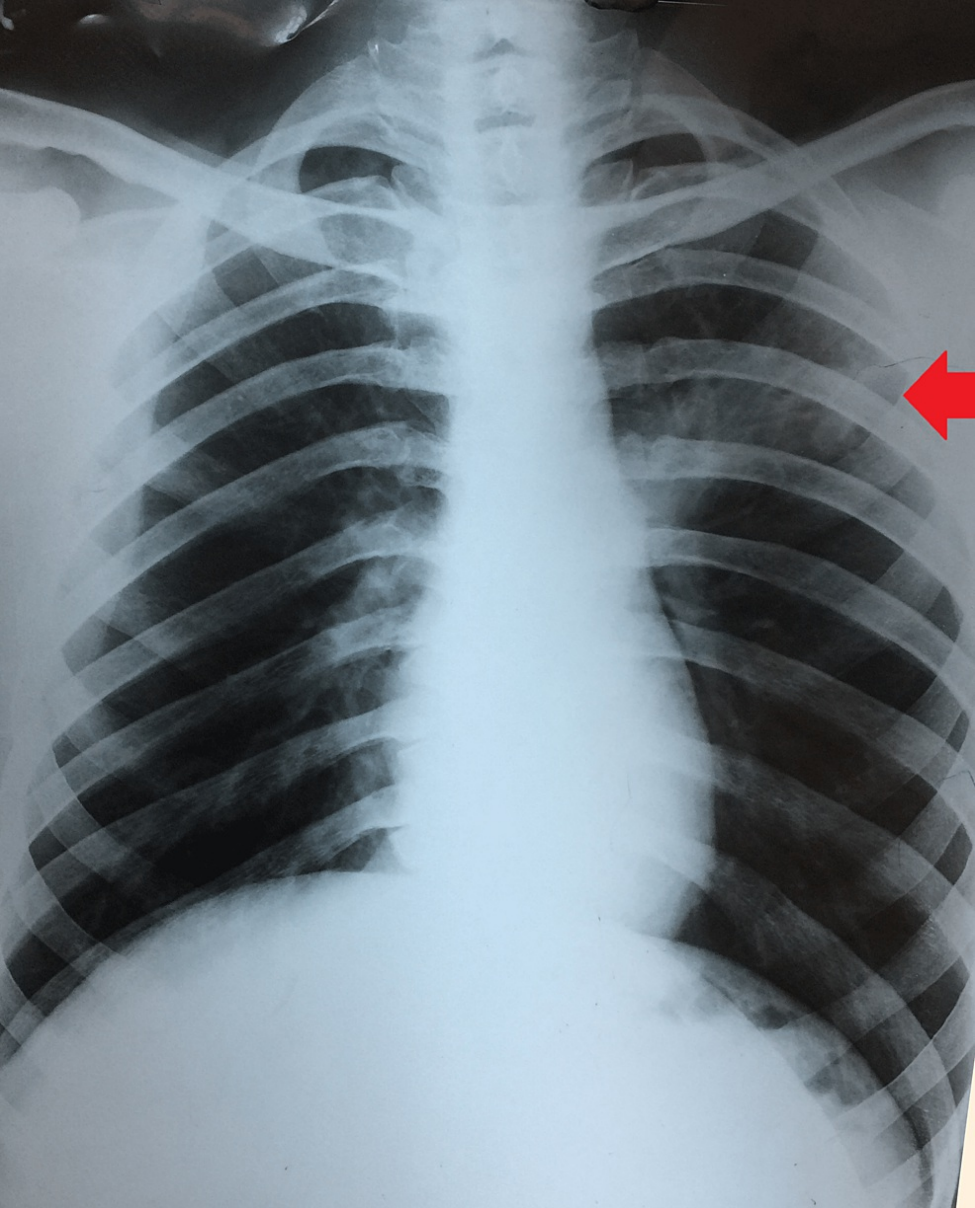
Chest radiograph (P-A view) showing left upper lobe consolidation P-A: Postero-anterior
